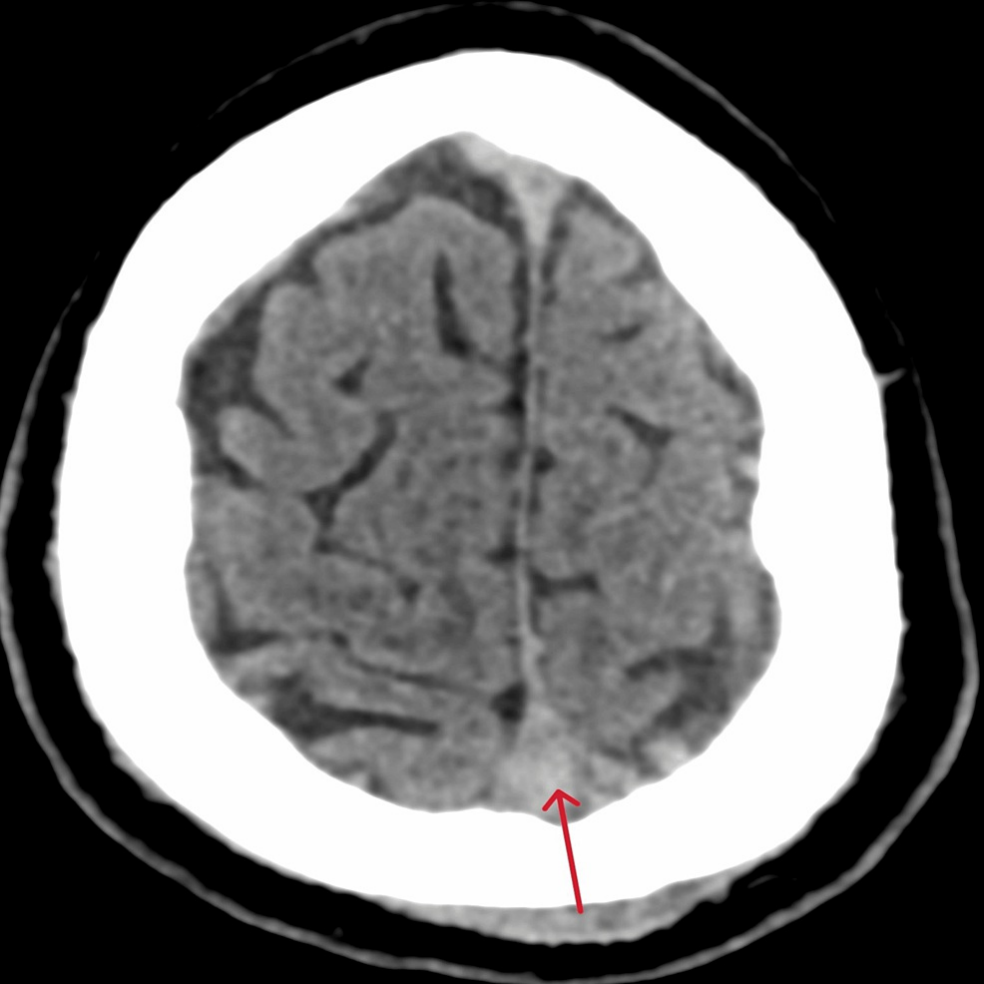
Hounsfield Unit value of non-contrast computed tomography of brain in cerebral venous sinus thrombosis Arrow shows hyperdense superior sagittal sinus with Hounsfield Unit value of 71.3.
